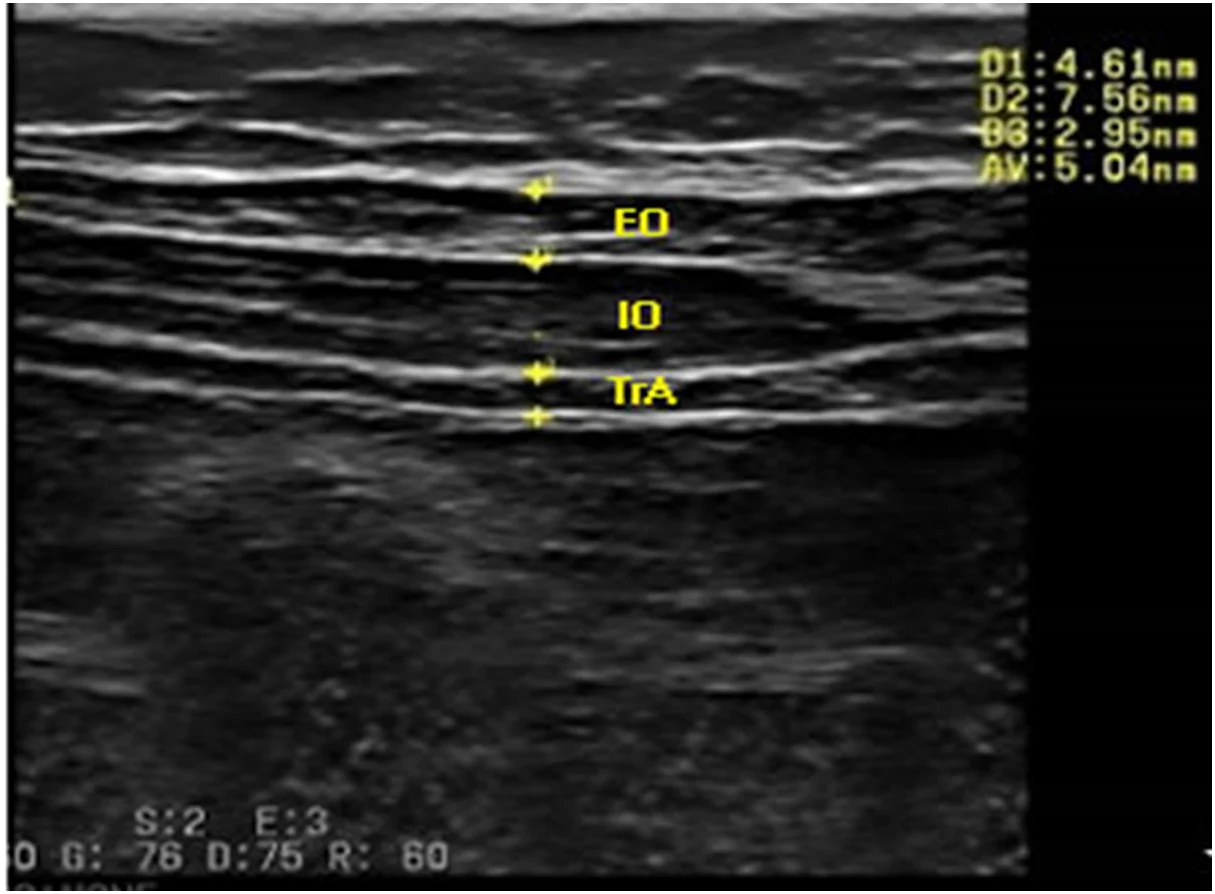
Ultrasound image of abdominal muscles at rest. Created by the authors. Published under CC BY 4.0.
